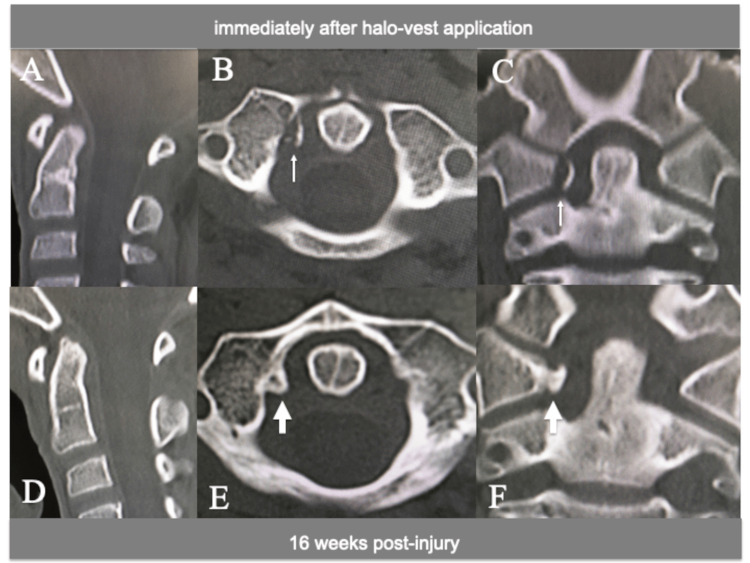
CT images immediately after halo-vest application and 16 weeks post injury CT showing (A, B, C) no increase in the displacement of the avulsed bone fragment immediately after halo vest application (arrows) and (D, E, F) solid fusion with the C1 lateral mass at 16 weeks post injury (large arrows).
